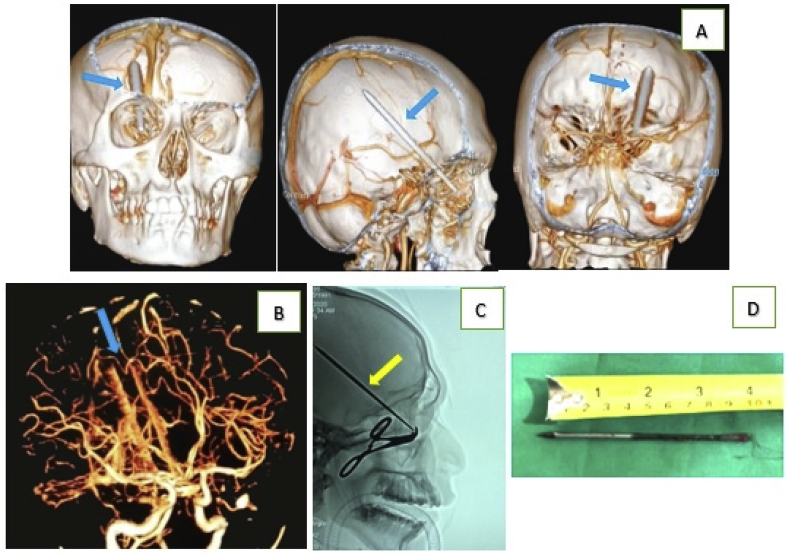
A). 3D CT (anterior, lateral, and posterior) showing the passage of the arrow through the right orbital roof into the ipsilateral cranium (arrows). B). CT angiography documented that there was no injury to the main vascular brain structures (arrows). C). C-arm guidance to remove the arrow (arrows). D). A metal arrow 10 cm in length.
